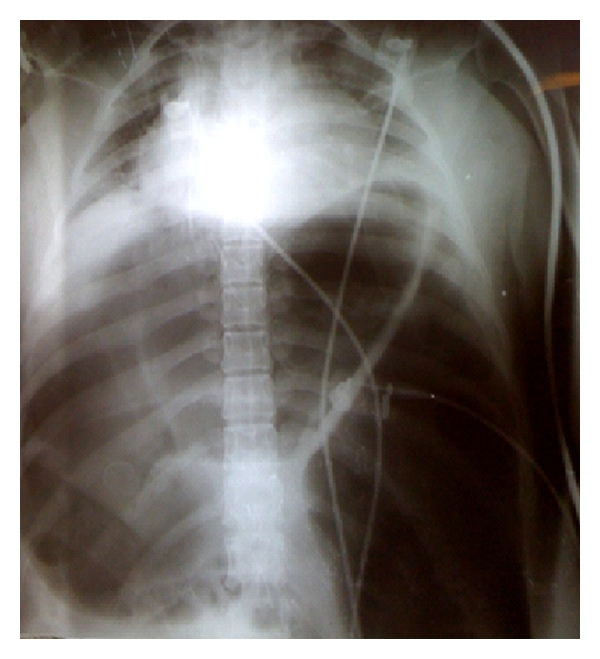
Plain radiography of the chest and abdomen showing severe distension of the transverse and sigmoid colon leading to a lung collapse.
